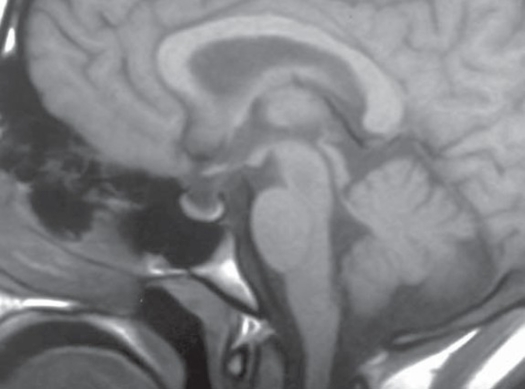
Sagital MRI T1 weighted image of the brain and sella showing features of increased intracranial pressure in a 7-year-old boy, partial empty sella syndrome.
